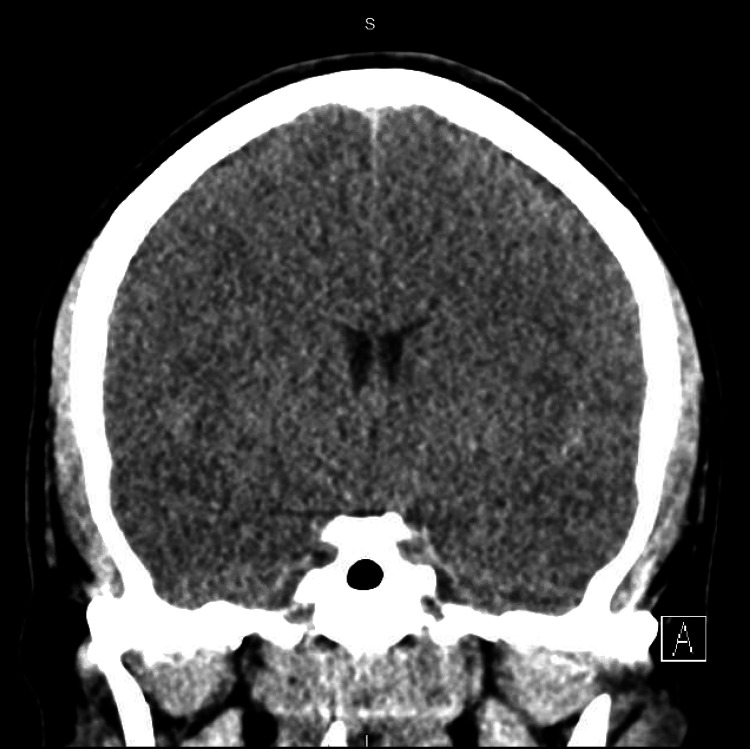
CT Head on presentation demonstrating loss of definition of the ventricular grey-white junction. The sulci are effaced as is the ventricular system. These findings are consistent with diffuse cerebral edema
